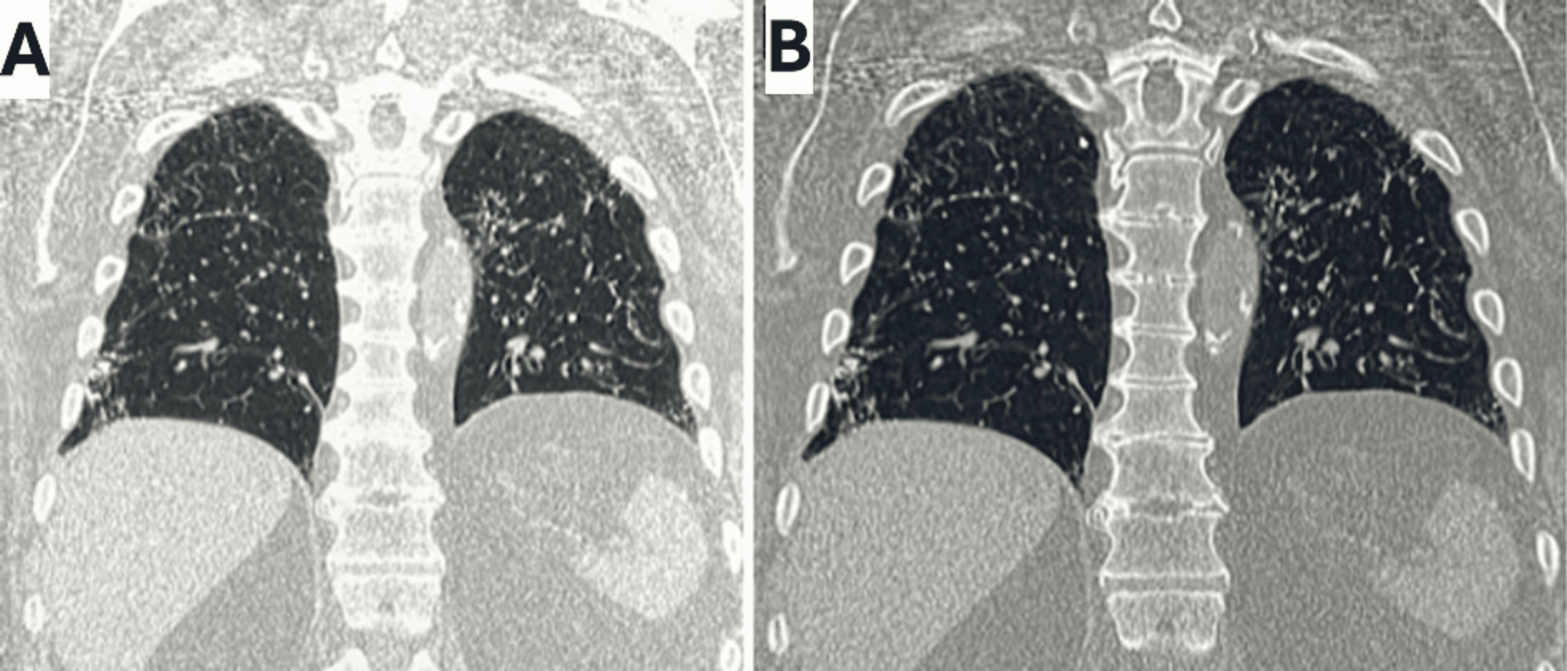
HRCT of the chest (coronal view) showing lung window (A) and bone window (B). Reveals extensive peripheral, bibasilar linear reticulations, interstitial thickening, and subpleural cystic changes consistent with advanced pulmonary fibrosis. Once again, branched calcified densities seen more prominently in the bone window (B). HRCT: high-resolution computed tomography
